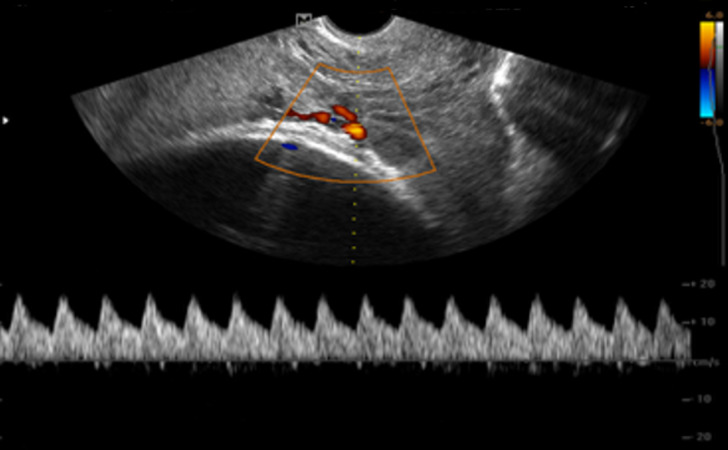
transvaginal ultrasound with pulse wave Doppler showing arterial waveform in fetal vessel
